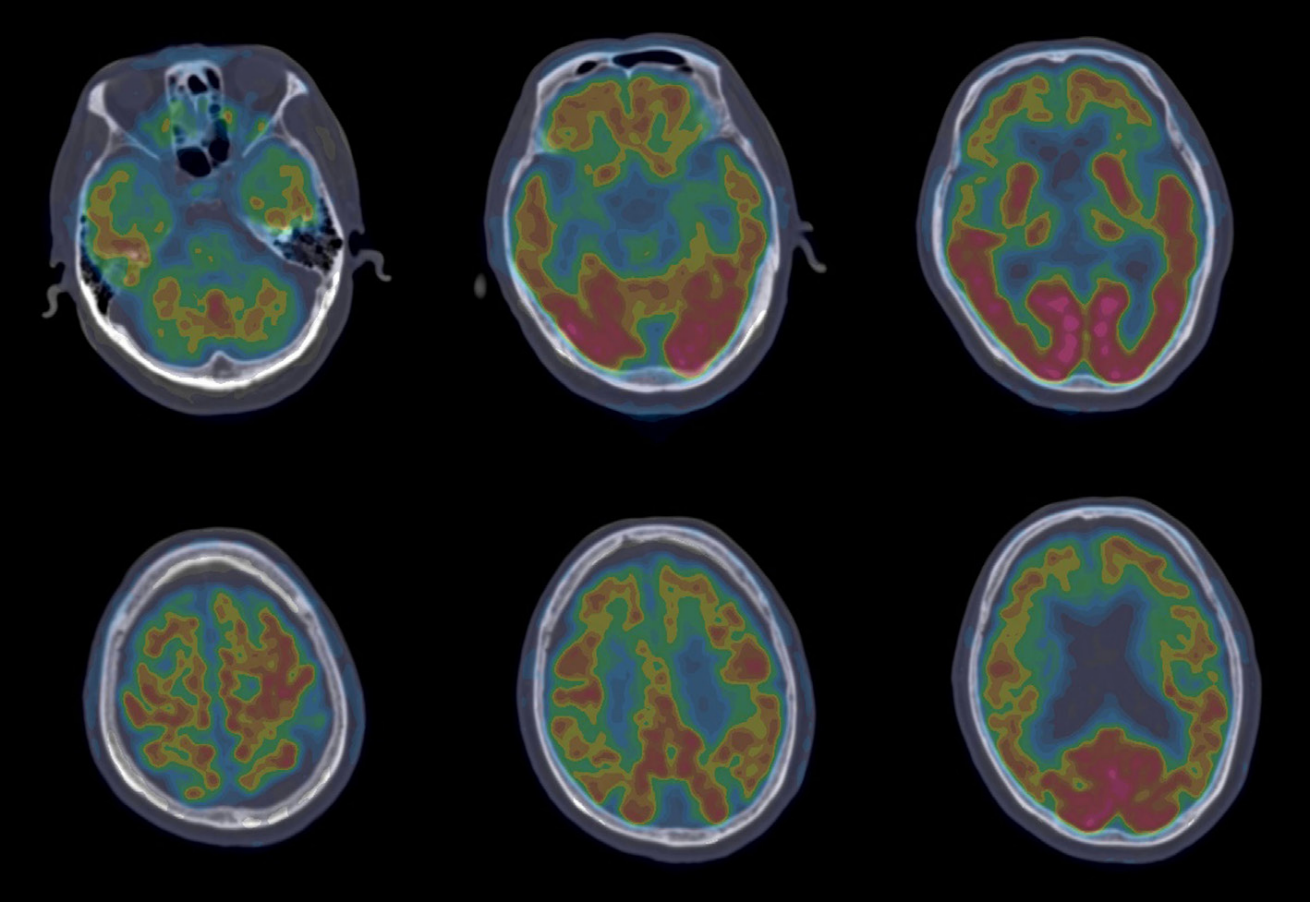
18F-FDG-PET revealed hypometabolism of the bilateral frontal, temporal, and parietal lobes that was more prominent on the frontal region.
